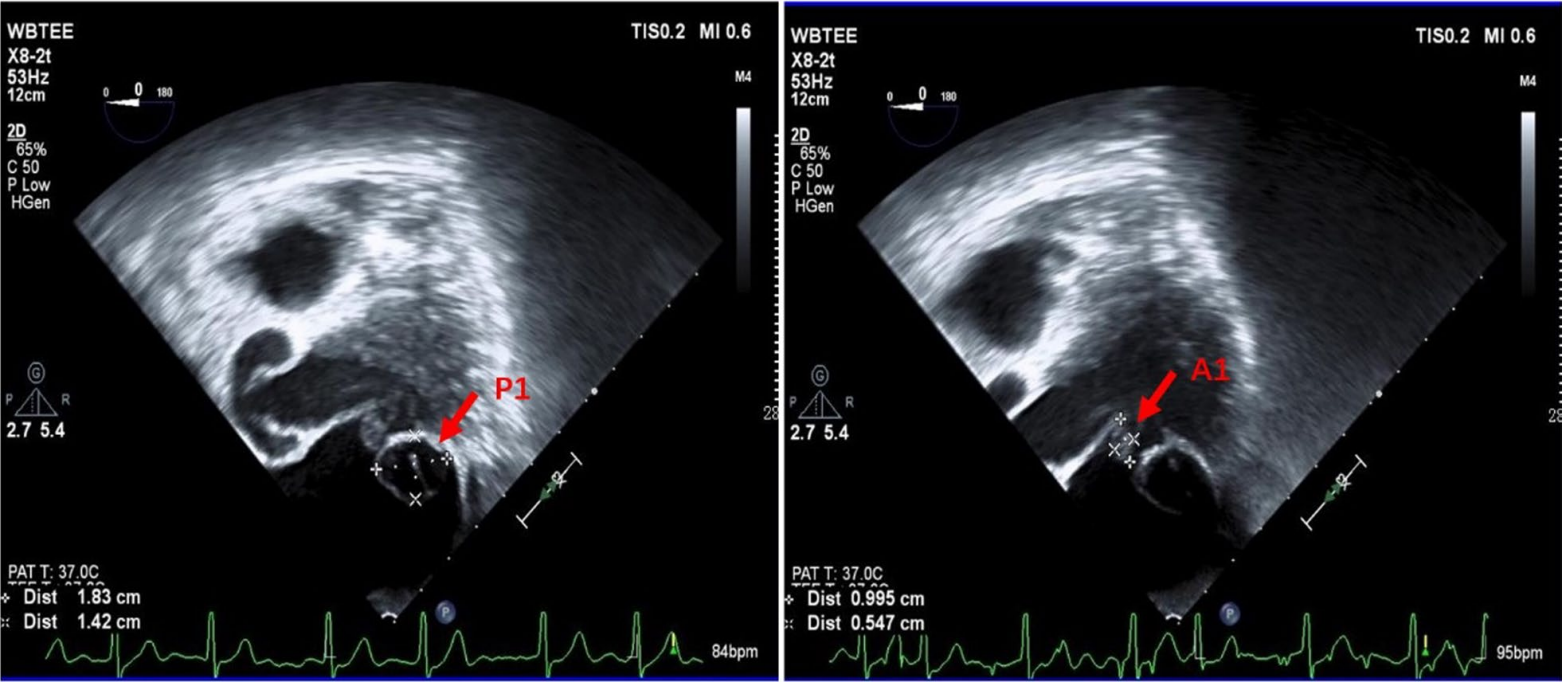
Transesophageal echocardiography showed that multiple cystic structures with visible separation were on the mitral valve leaflets. The larger one was located in the P1 area, about 18.3 × 14.2 mm in size, and smaller in the A1 area about 9.9 × 5.4 mm
